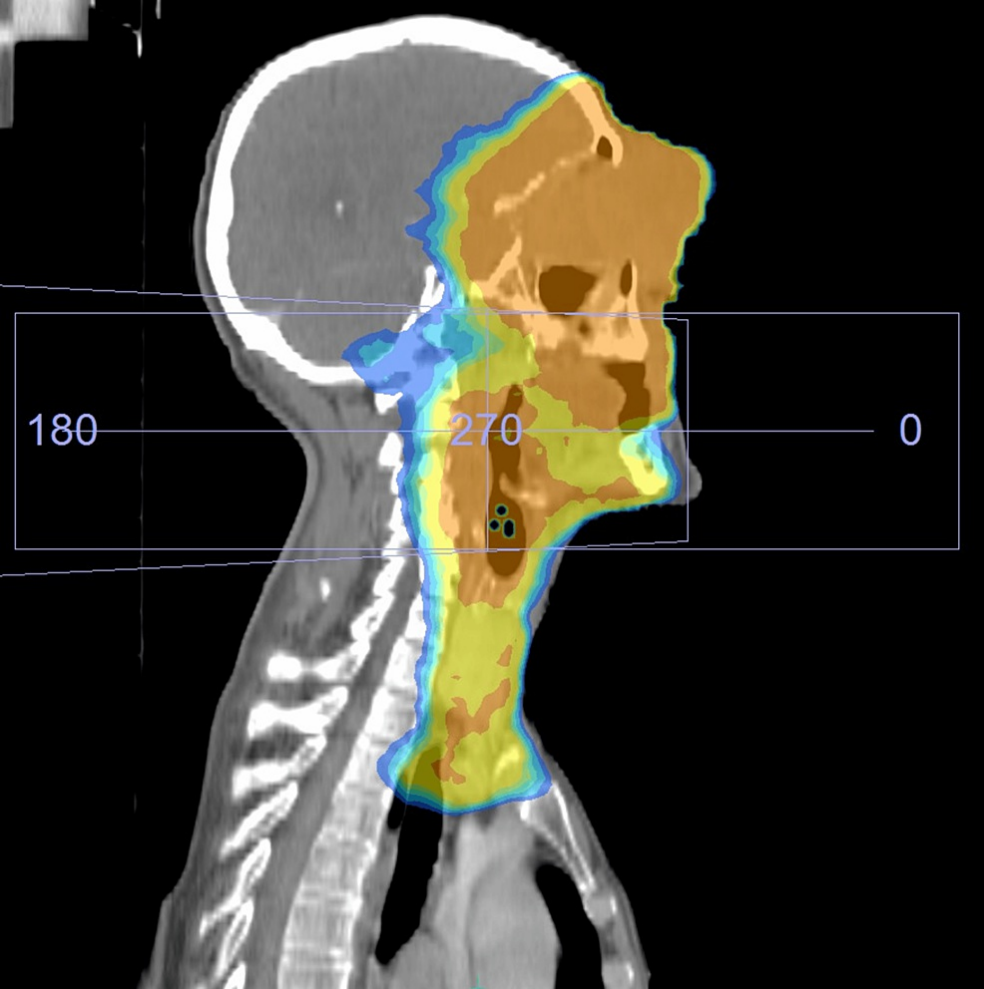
Radiotherapy isodose curve (sagittal view): the planning CT scan with 60 Gy (in yellow) and 70 Gy (in orange) isodose lines.
